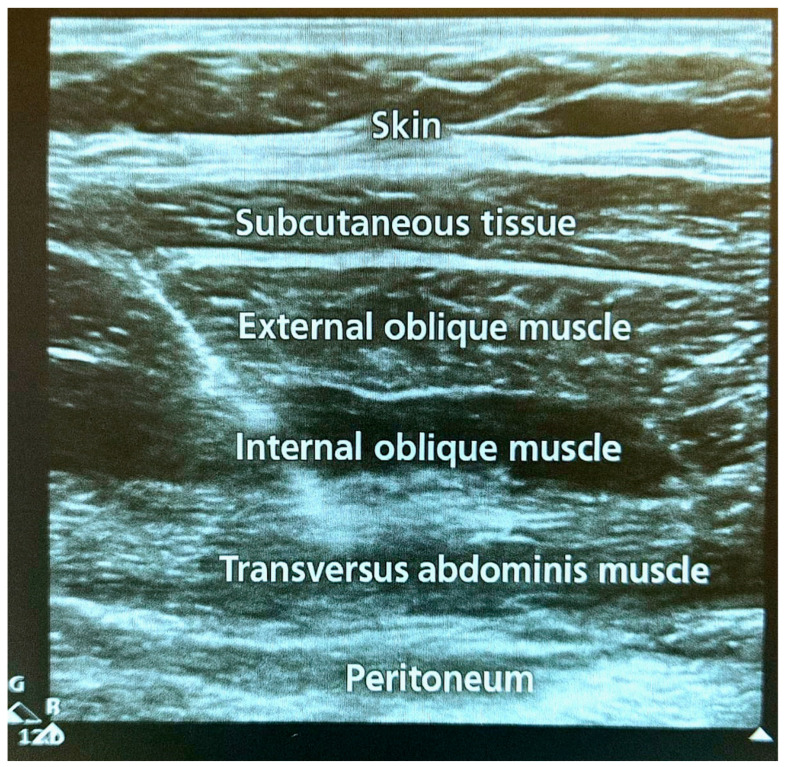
Ultrasound-guided insertion of the needle for transversus abdominis plane block to deliver local anesthesia.
